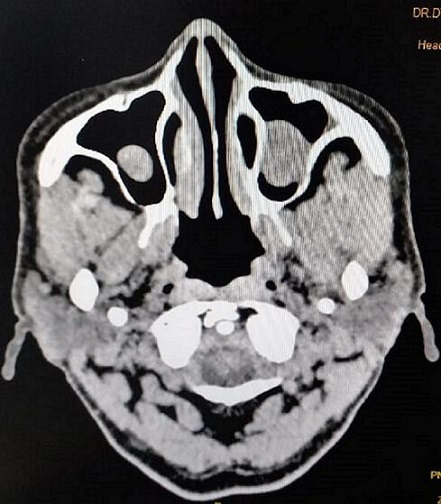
Case 3. CT PNS: mucosal thickening in the maxillary and ethmoidal sinuses.
